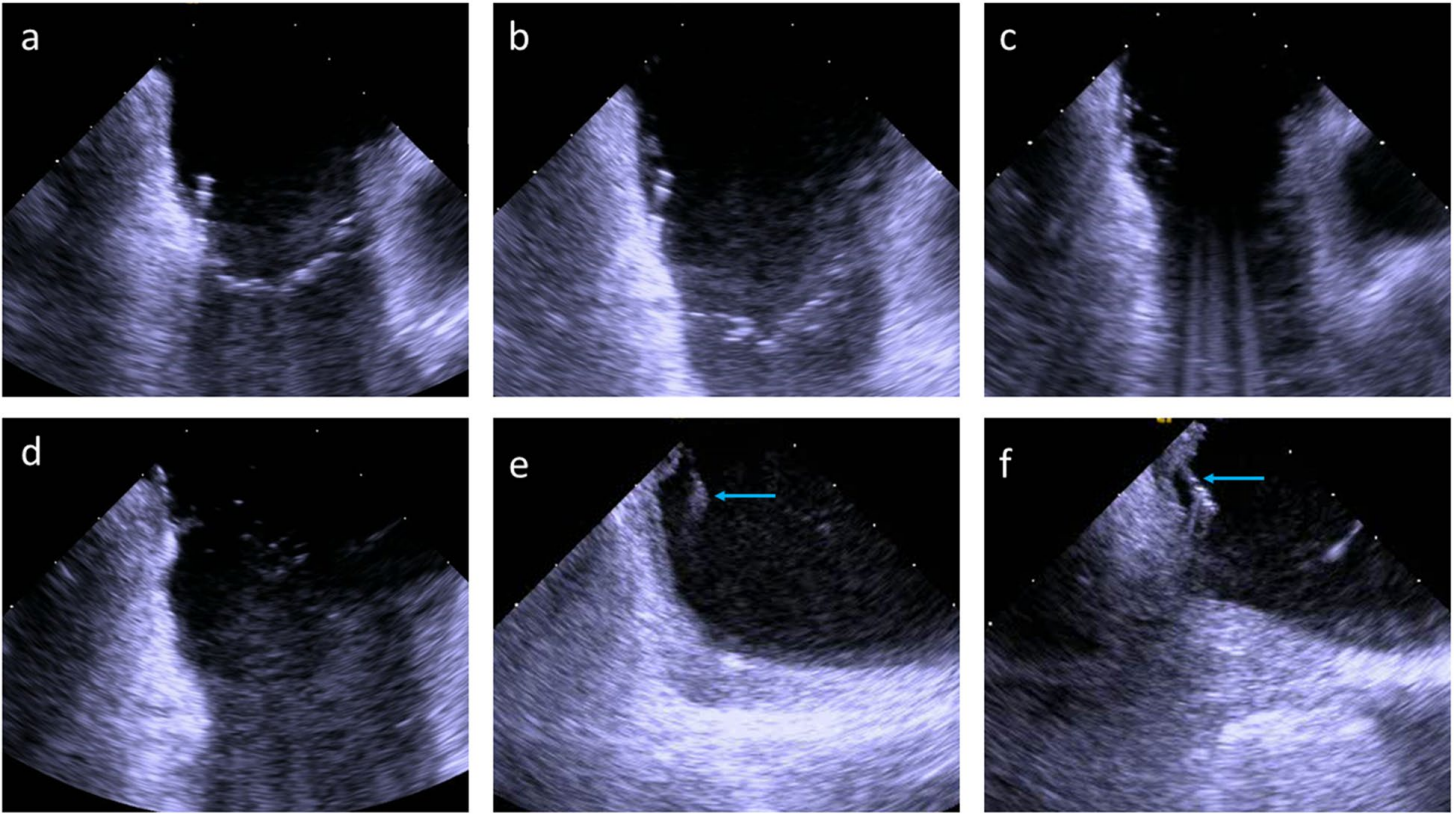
a–d Typical ablation planes with CTI on the left side. The ablation catheter is dragged from the tricuspid valve ring to the inferior caval vein, maintaining the catheter on the echocardiographic plane to achieve the best possible line; e insufficient contact of the ablation catheter tip (blue arrow) to the target tissue due to a prominent Eustachian ridge; f corrected tissue contact by inversion of the ablation catheter (blue arrow)
